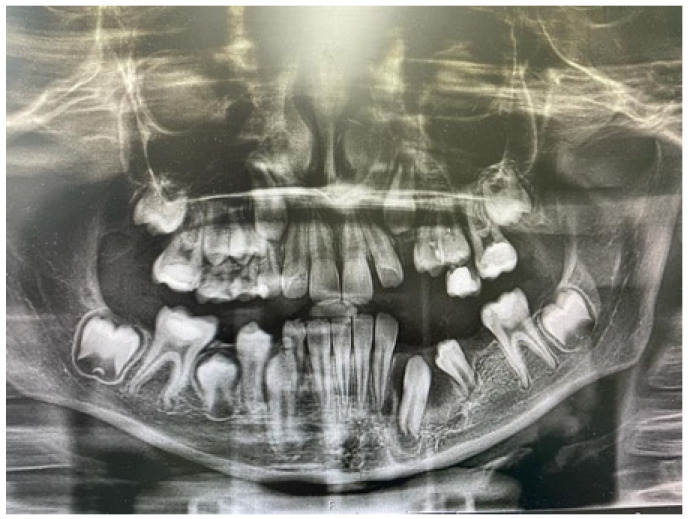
Three-month postoperative panoramic radiograph showing a significant reduction of the lesions. The decompression tubes were removed.
